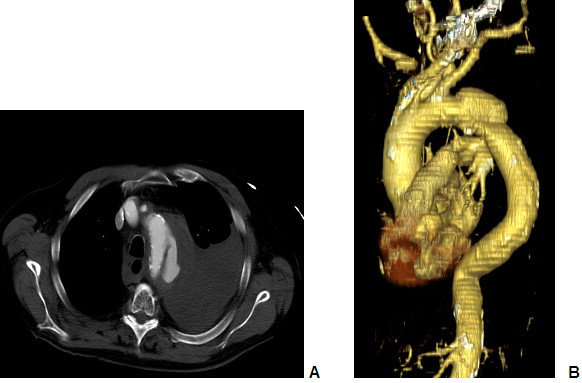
A+B: Computed tomography scans obtained at admission ruptured dissection type B and extensive left-sided hematothorax.
